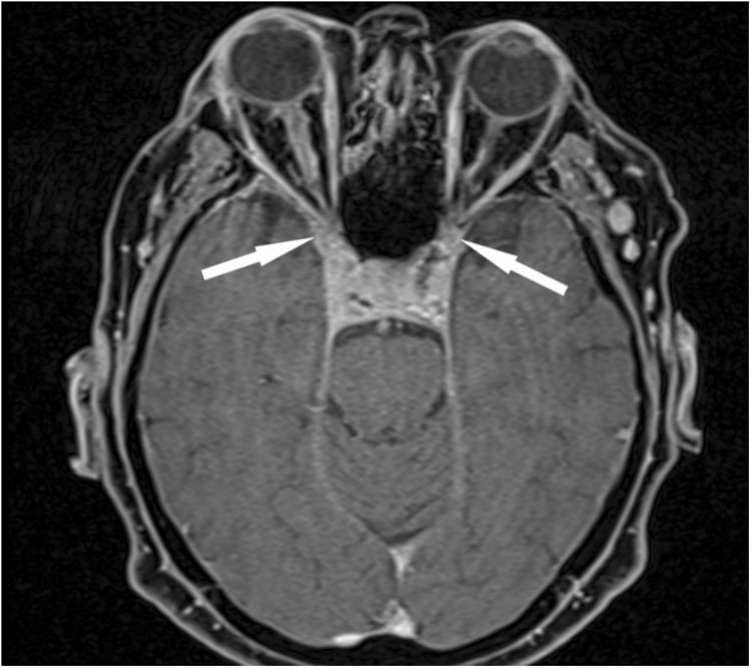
Initial MRI scan, axial plane, after contrast medium administration. White arrows indicate the infiltration that encompasses superior orbital fissures
